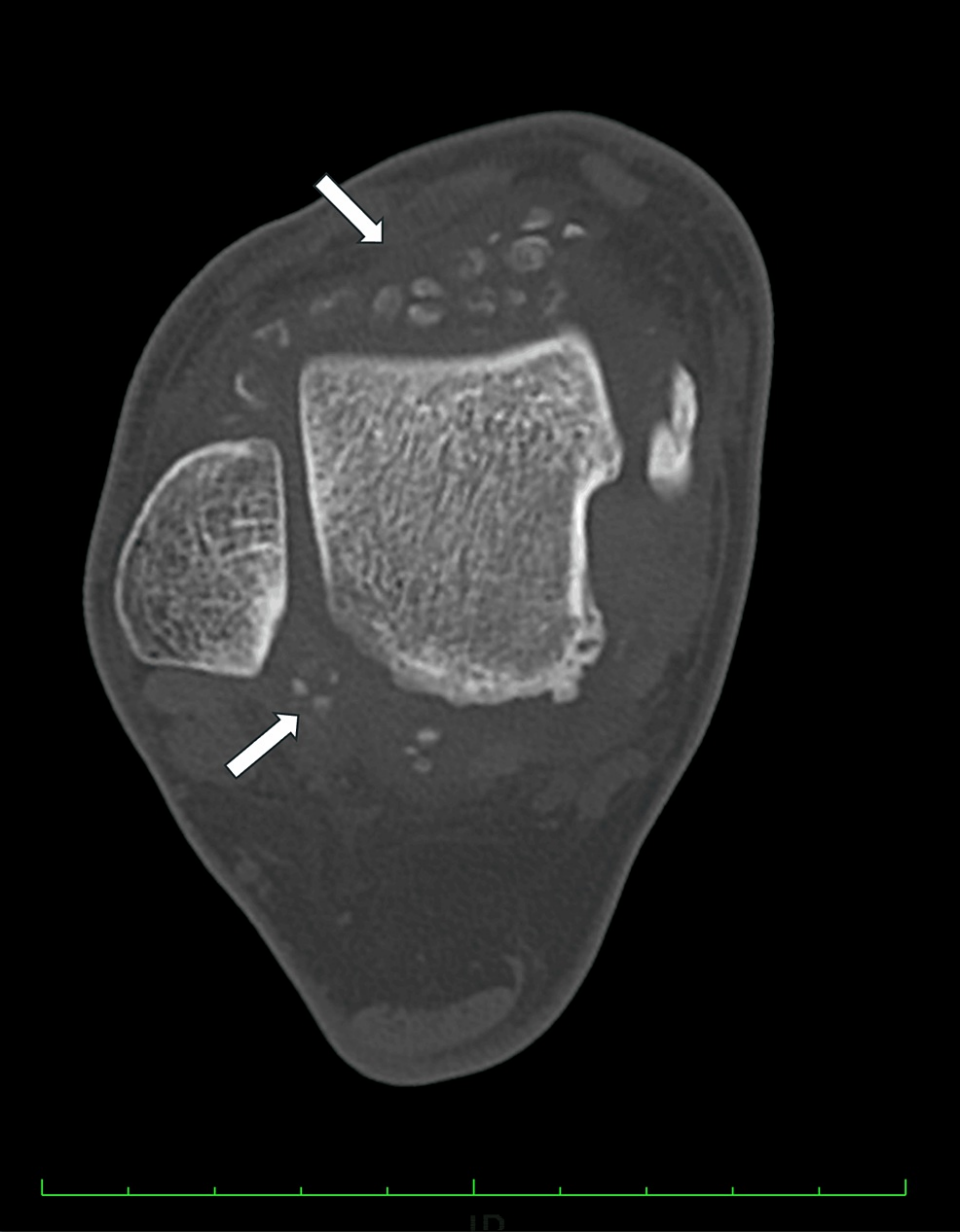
Preoperative axial CT of the right ankle.
